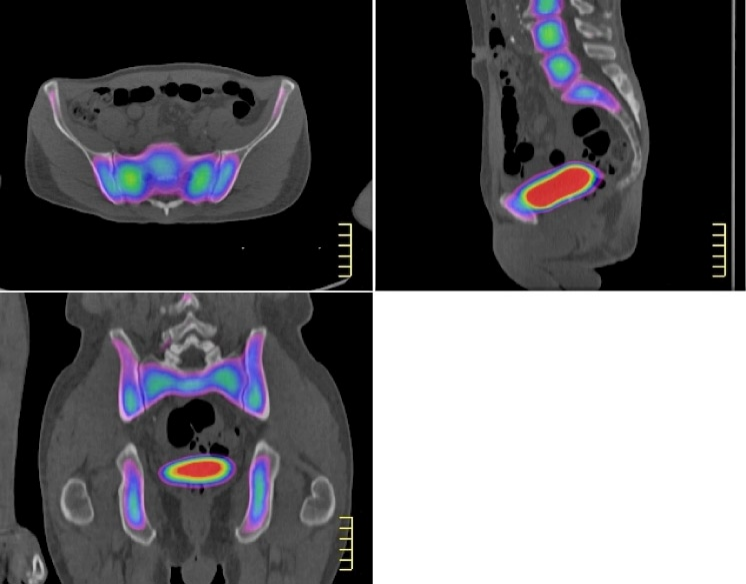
Axial, sagittal, and coronal views of the registered FLT PET and CT images for one subject. Color wash represents increasing FLT uptake from purple to red and blue approximately equal to SUV 2.
